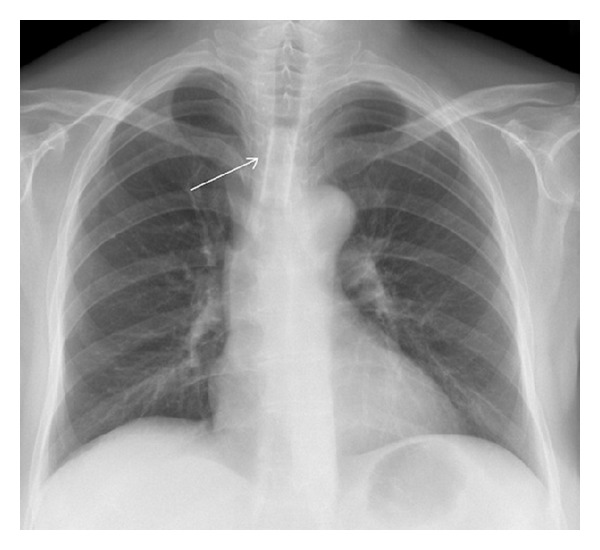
Chest X-ray with tracheal stent in place.
